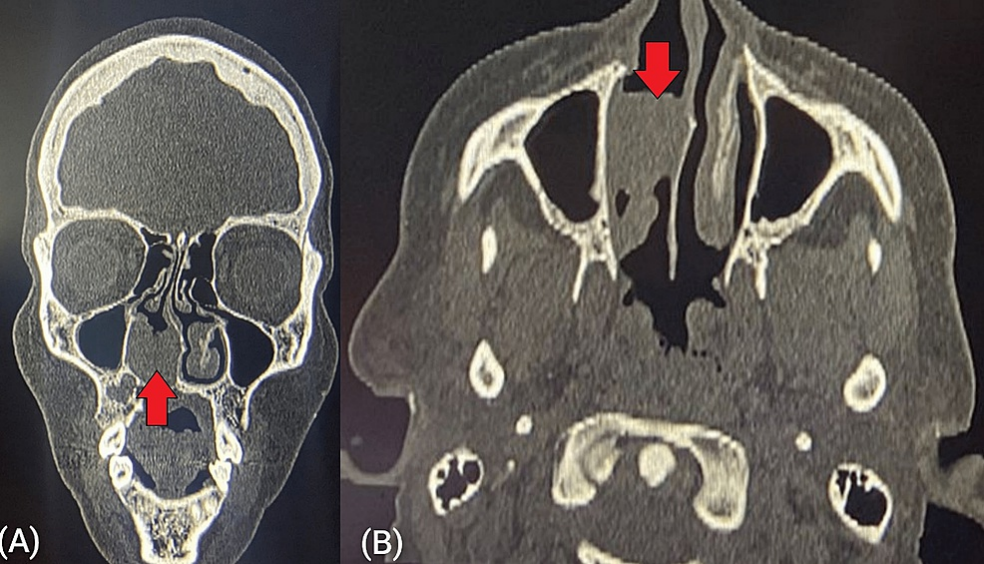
(A) CT image (coronal section) of pleomorphic adenoma of the nasal septum; (B) CT image (axial section) of pleomorphic adenoma of the nasal septum CT: computed tomography
